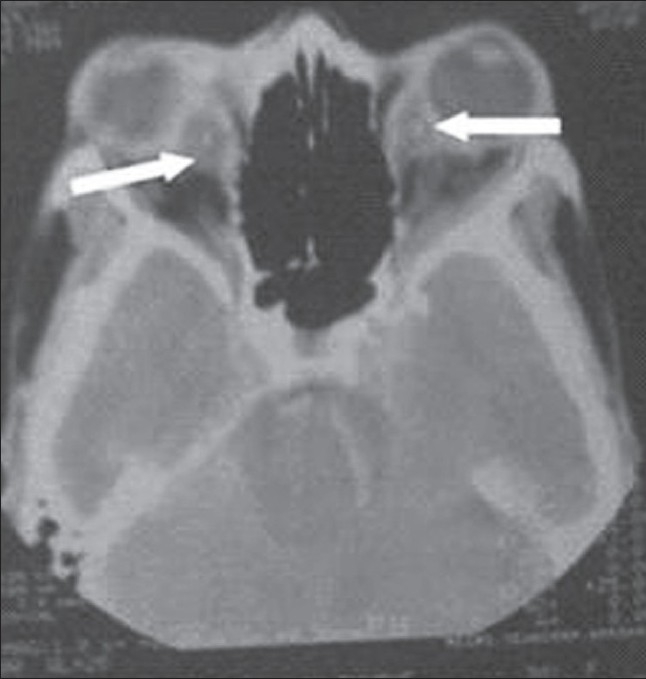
CT Image showing subdural hematoma in same patient
